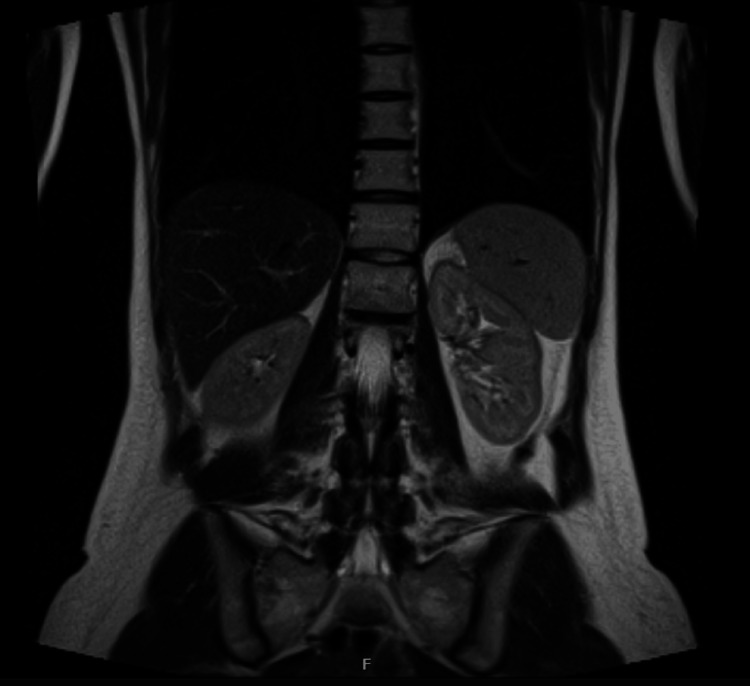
MRI of the bilateral adrenal gland (coronal section): no adrenal pathology MRI: magnetic resonance imaging
